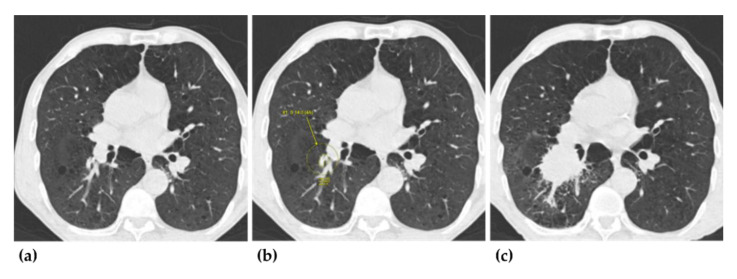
Missed lung cancer in the right lower lobe. (a,b) During the computed tomography (CT) examination of the first screening round (T0), the lesion was smaller in size and was missed by the interpreting radiologist (as shown in image a), but the deep-learning based computer-aided diagnosis system (DL-CAD) detected the missed lung cancer (as shown in image b) at the same round of CT screening. (c) The CT examination of the second screening round (T1) showed a large irregular mass, and the lesion was confirmed as lung cancer (adenocarcinoma).
